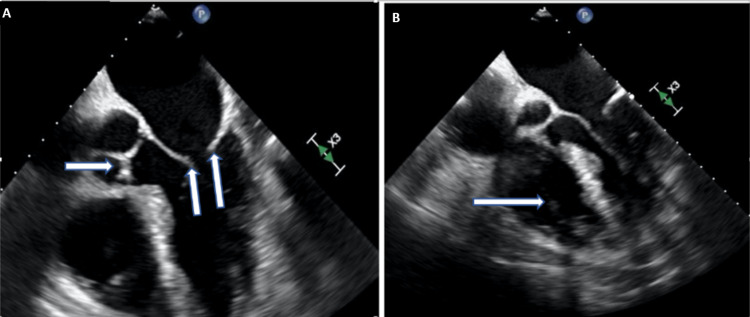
Transesophageal echocardiogram (four-chamber views) 2A. This view shows no evidence of vegetations involving mitral valve leaflets (vertical arrows) or aortic valve cusps (horizontal arrow). 2B. This view displays no obvious vegetations over the right ventricular pacemaker lead (arrow)
